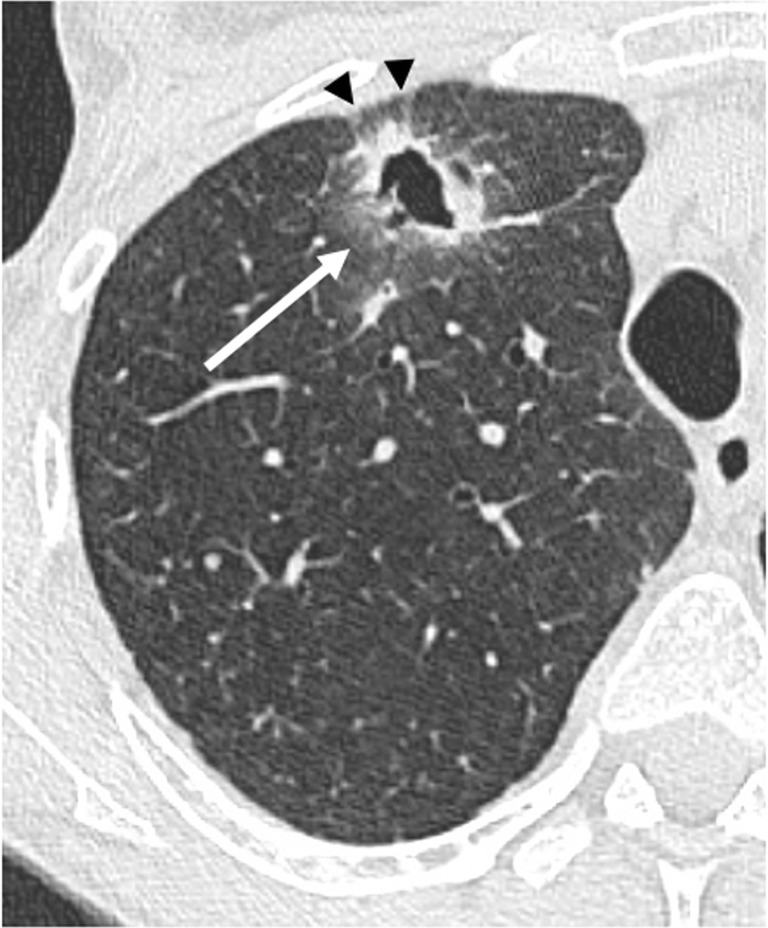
Primary epidermoid lung carcinoma. Asymptomatic, cavitated nodule on follow-up chest X-ray. Cavitated nodule with spiculated margins (*arrow*) and pleural traction (*arrowheads*)
